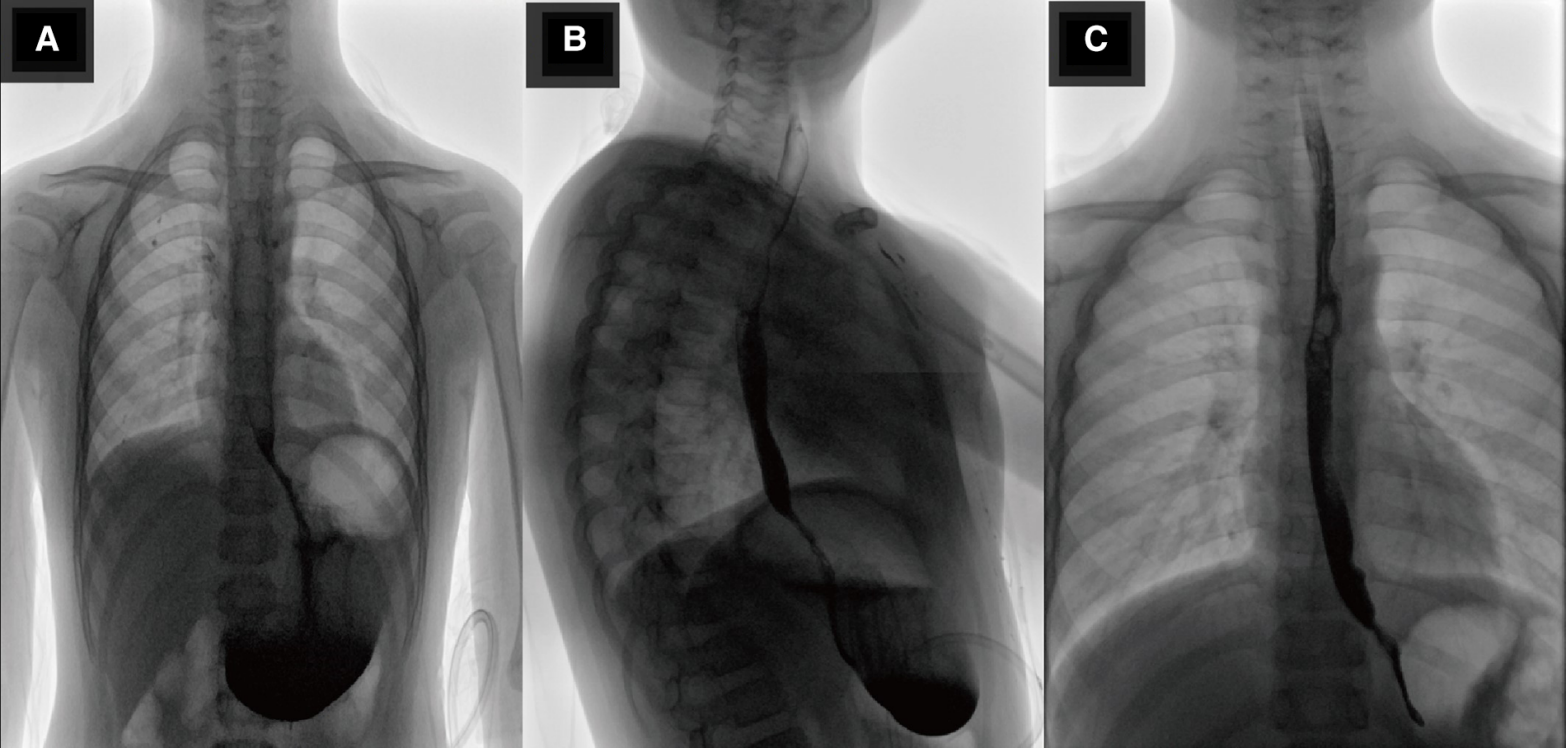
(A,B) Show the radiological investigation of the child: positive and lateral upper gastrointestinal radiographs reviewed 10 days after surgery, showing good recovery. (C) Shows the radiological investigation of the child: re-examination of the upper gastrointestinal tract contrast study 2 months after surgery, showing good recovery.
